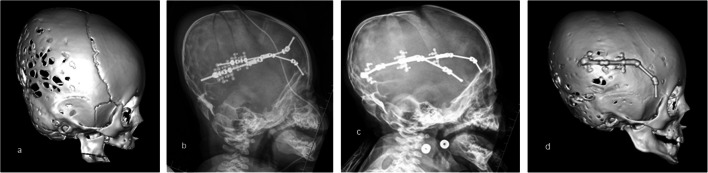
A Preoperative 3D CT image; b postoperative x-ray; c x-ray taken at the follow-up; d 3D CT image taken prior to removal of the devices, where ossification is visible
